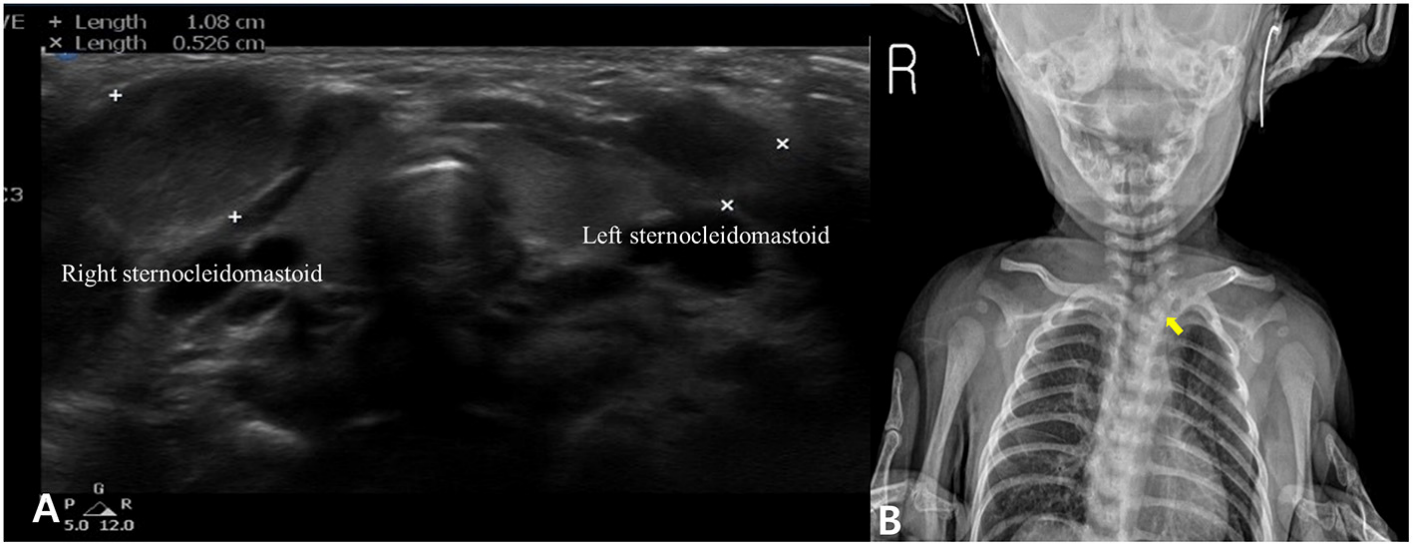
Ultrasonography showing diffuse enlargement and hyperechoic findings in the right sternocleidomastoid muscle (A). A congenital vertebral anomaly was suspected in the clavicle X-ray imaging (yellow arrow) (B).
